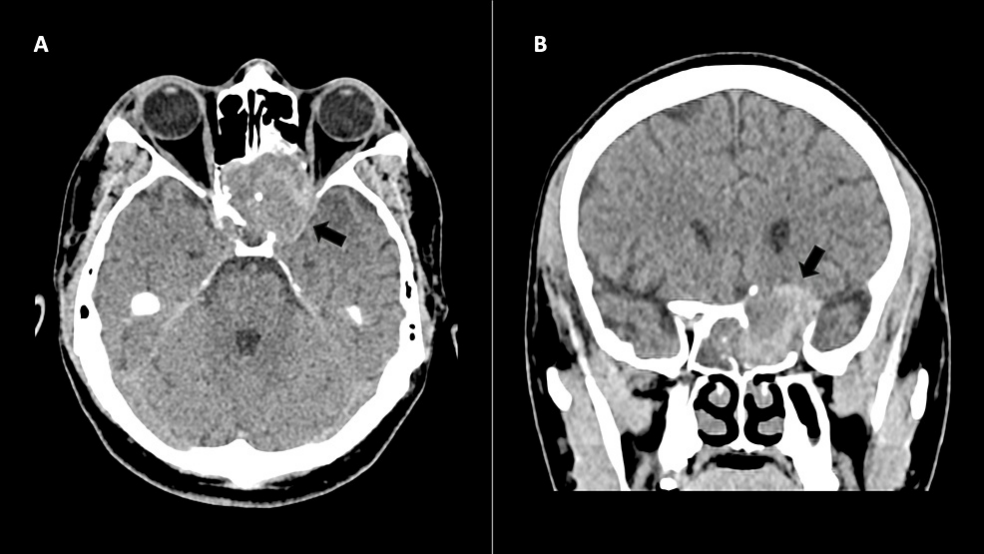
Skull CT. A: axial B, coronal. Heterogeneous mass (arrows) with hyperdense areas, located in the left sphenoid sinus, showing remodeling of the sinus walls, posterior ethmoid cells, medial wall of the left orbit, and a bone defect in the base of the skull (anterior cranial fossa and medial cranial fossa).
